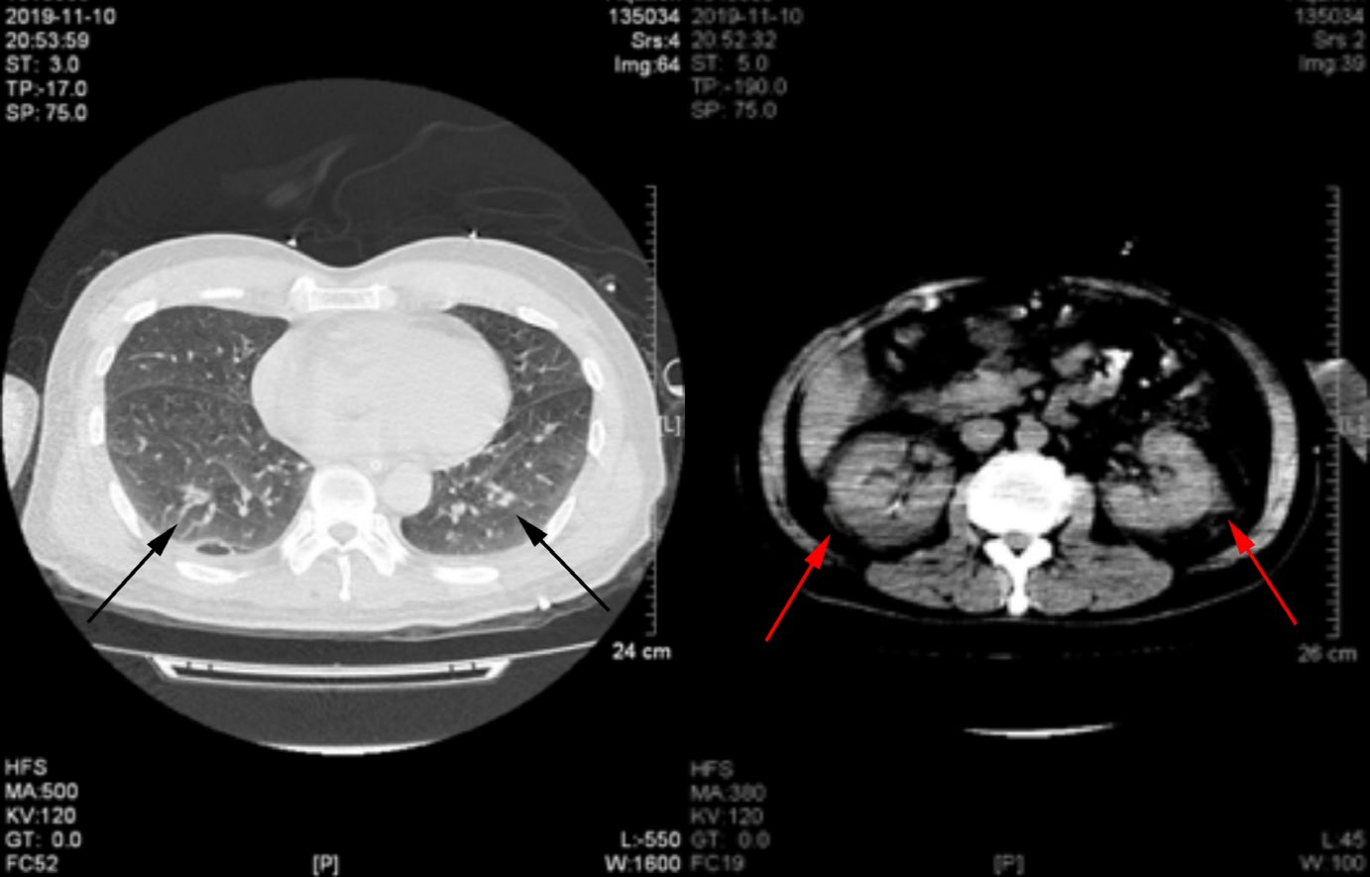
The chest and kidney CT on ICU admission, which suggested slight bilateral inflammation (left panel, black arrow) of lower lungs and perirenal inflammation (right panel, red arrow)
